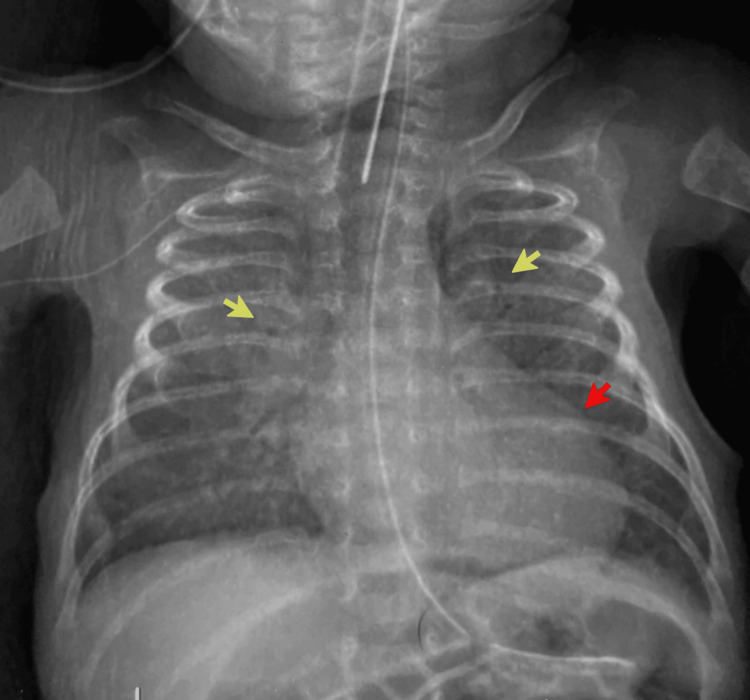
The pre-procedural anteroposterior radiograph demonstrates marked cardiomegaly (red arrow) with diffuse pulmonary overcirculation and perihilar haze (yellow arrow), suggestive of pulmonary venous congestion.
